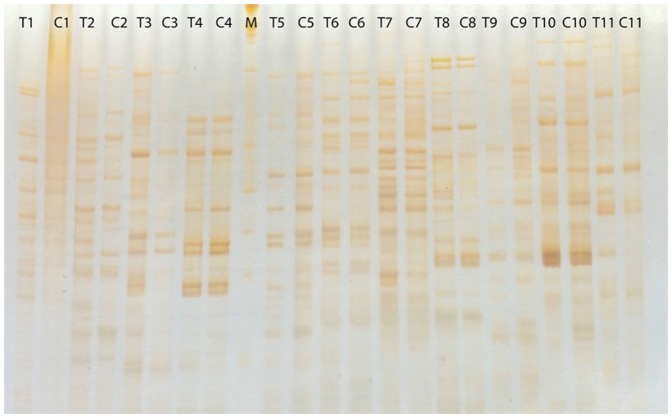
An example of TDFs in a polyacrylamide gel with 13 primer combinations included: EcoR1+AT, CC and Mse1+TG, CT, GC, GA, AG, GT, AT, TT, CG, GG.
